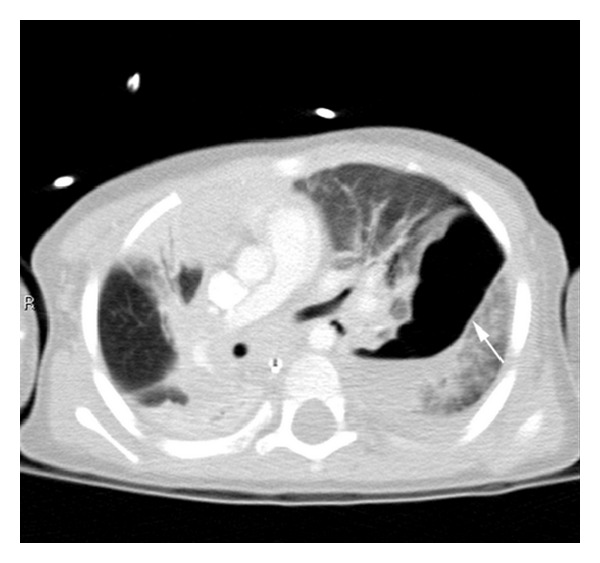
Axial view on CT chest. The pneumatocele measured approximately 6 cm (height) × 3 cm (width) × 4 cm (anteroposterior dimension).
